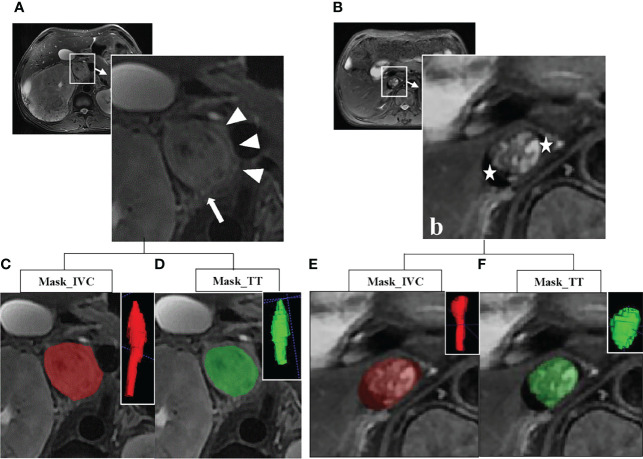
(A) Axial fat-suppression T2-weighted image (fsT2WI) in a 68-year old man with a clear cell renal cell carcinoma (cRCC) and an inferior vena cava (IVC) tumor thrombus (TT) with wall invasion. Subjective features of complete occlusion of the IVC lumen, the irregular margin of the TT (arrow), thickened IVC wall (arrow and triangle), and abnormal signal of the IVC wall (arrow) can be found. (B) Axial fsT2WI in a 76-year old woman with cRCC and a TT in IVC without wall invasion. The crescent-shaped black areas on the laterodorsal aspect of IVC stand for flow void (star). (C–F) Examples for annotation of IVC and TT. The red represents the mask of the IVC, and the green represents the mask of the TT. The three-dimensional (3D) volumes of interest (VOIs) are at the upper right corner.
